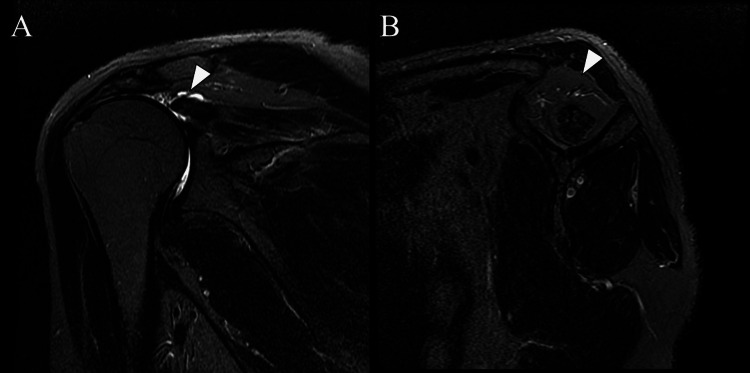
Preoperative magnetic resonance imaging of the right shoulder (A) Fat-suppressed T2-weighted image obtained in the oblique coronal plane shows massive rotator cuff tears at the level of the supraspinatus tendon; (B) Fat-suppressed T2-weighted image obtained in the sagittal plane showing advanced fatty infiltration of the supraspinatus muscle.
